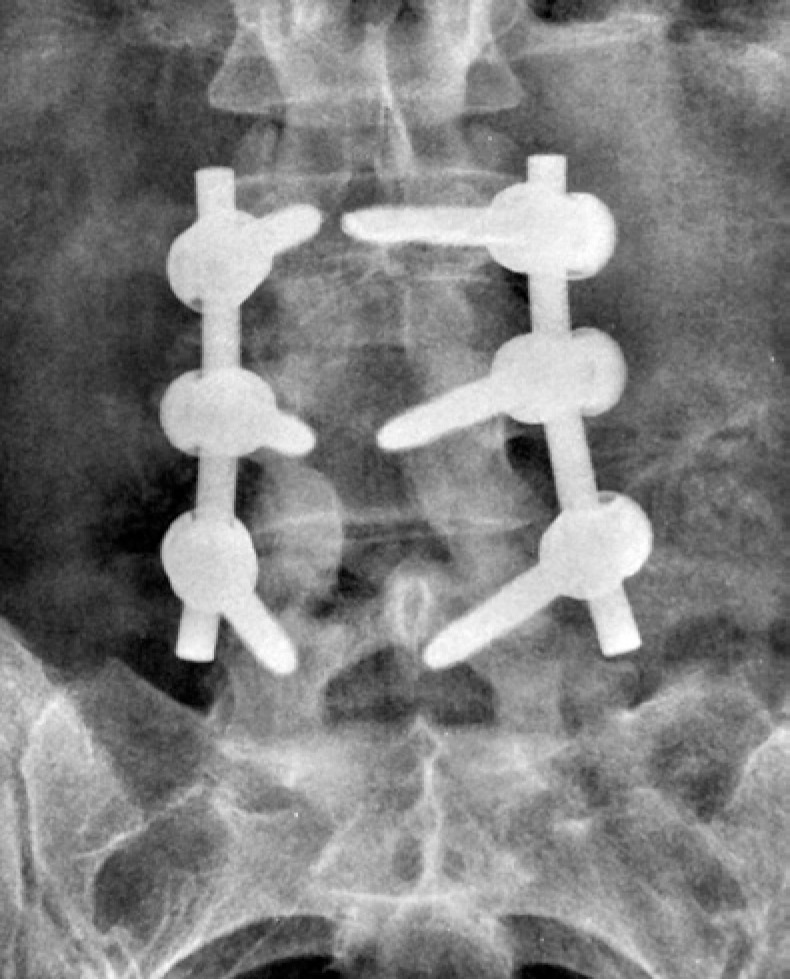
Anteroposterior radiography of the lumbosacral spine displaying doubtful posterolateral fusion, due to the presence of metal stems superimposed on the intertransverse space
